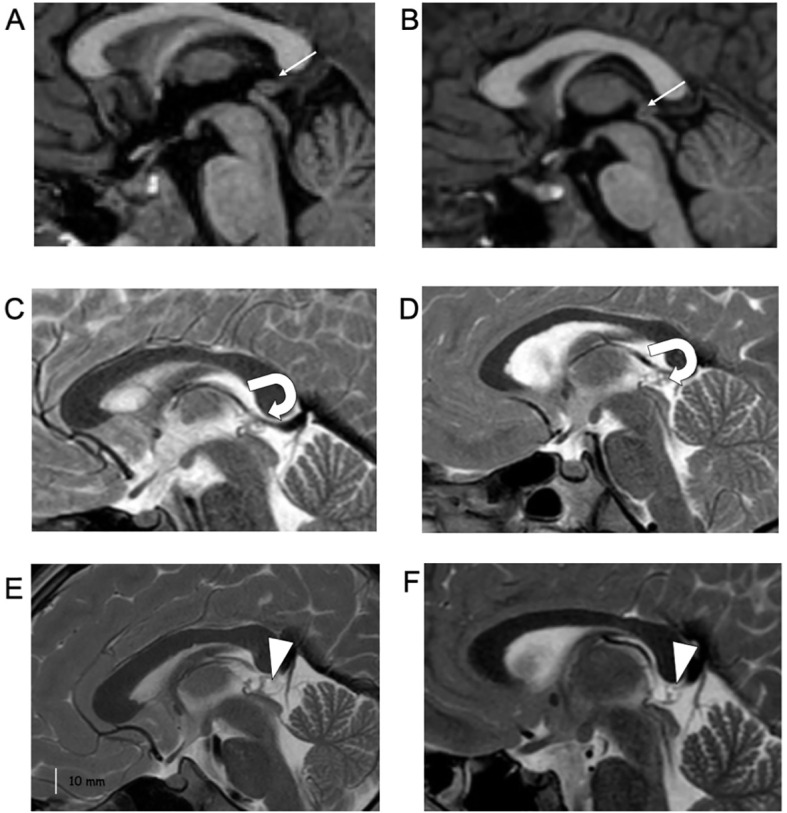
Comparative MRI characteristics of pineal gland in prepubertal controls and in girls with central precocious puberty. (A, B) Sagittal 3DMPRAGE images show the appearance of a normal pineal gland without pineal cyst in (A) prepubertal control and (B) CPP girl, respectively (arrows). (C, D) Sagittal T2 images show a microcystic pineal gland (curved arrow) in (C) prepubertal control and (D) CPP girls. It is characterized by small, well-defined hyperintense cystic components within the glandular parenchyma. (E, F) Macrocystic pineal gland in (E) prepubertal control and (F) CPP girl, respectively (arrow head). It is defined by larger fluid-filled cavities with thin smooth walls. A 10-mm scale bar is provided for reference.
